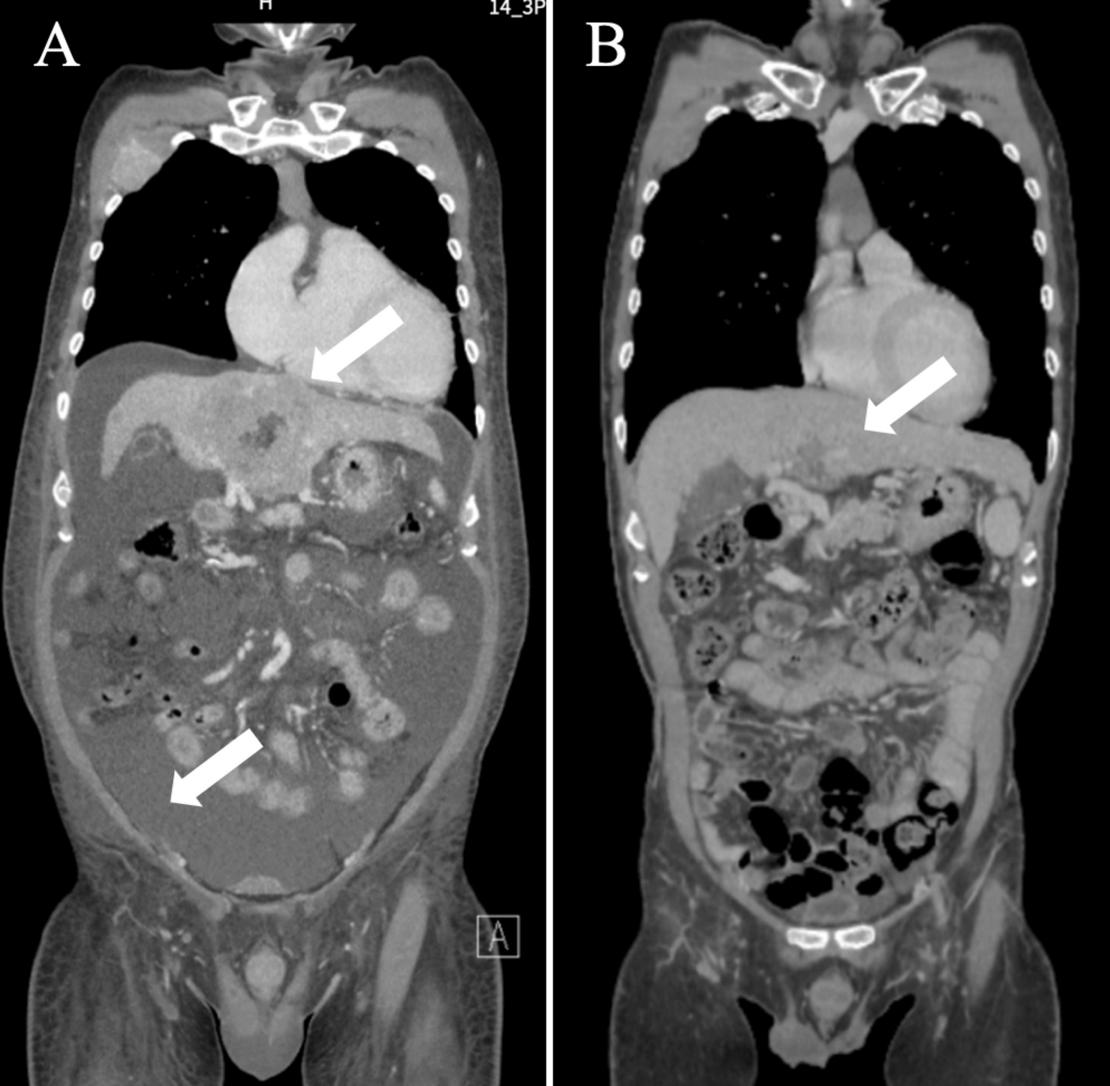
Initial status of the patients at administration, as shown by a CT scan of the coronal plane. CT: computed tomography.
